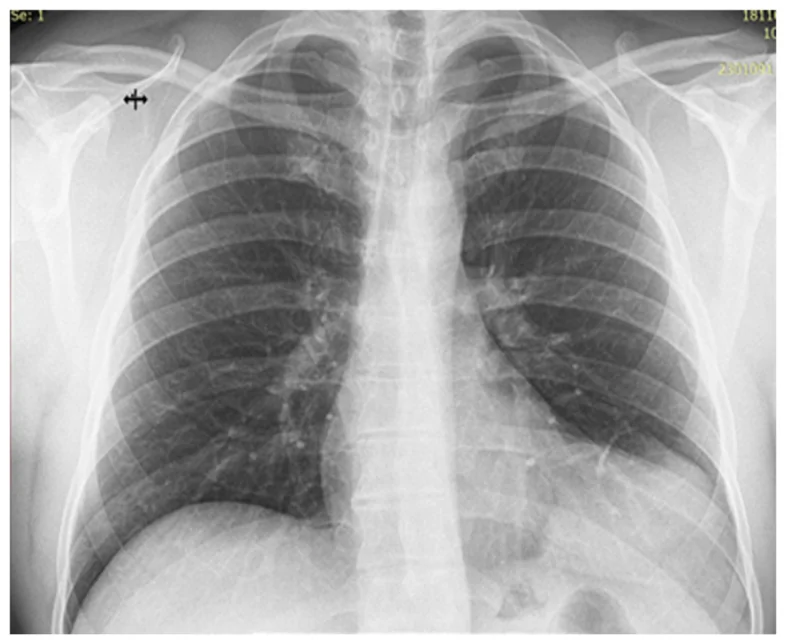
Initial posteroanterior chest radiograph demonstrating a well-defined opacity in the lower left hemithorax.
